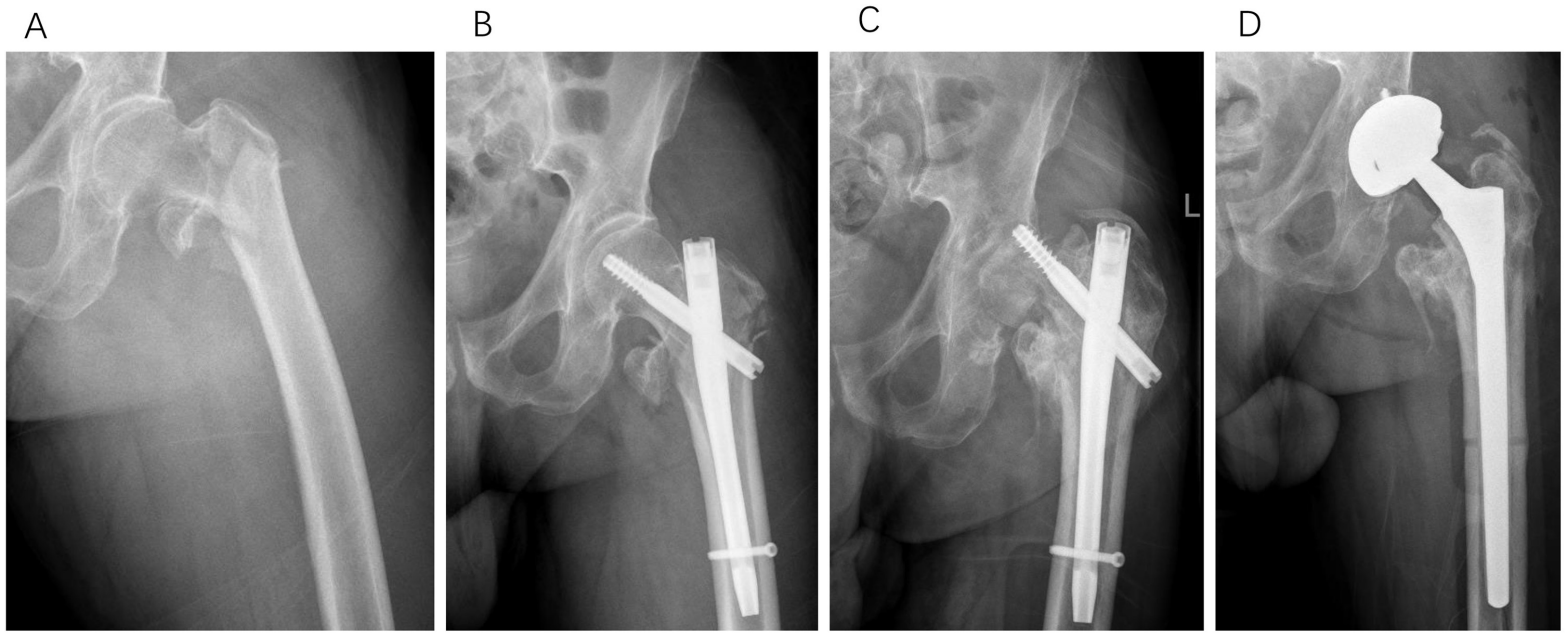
Type III, a 67-year-old male patient underwent Gamma nail fixation for a left intertrochanteric femoral fracture. Thirteen months post-operatively, radiographic evaluation revealed screw cutout and femoral head necrosis. In the subsequent revision surgery, we performed total hip arthroplasty. (A) Pre-operative anteroposterior X-ray of the injured hip. (B) Anteroposterior X-ray immediately following initial Gamma nail fixation. (C) Anteroposterior X-ray obtained 13 months after initial Gamma nail fixation. (D) Anteroposterior X-ray immediately following the revision surgery with total hip arthroplasty.
